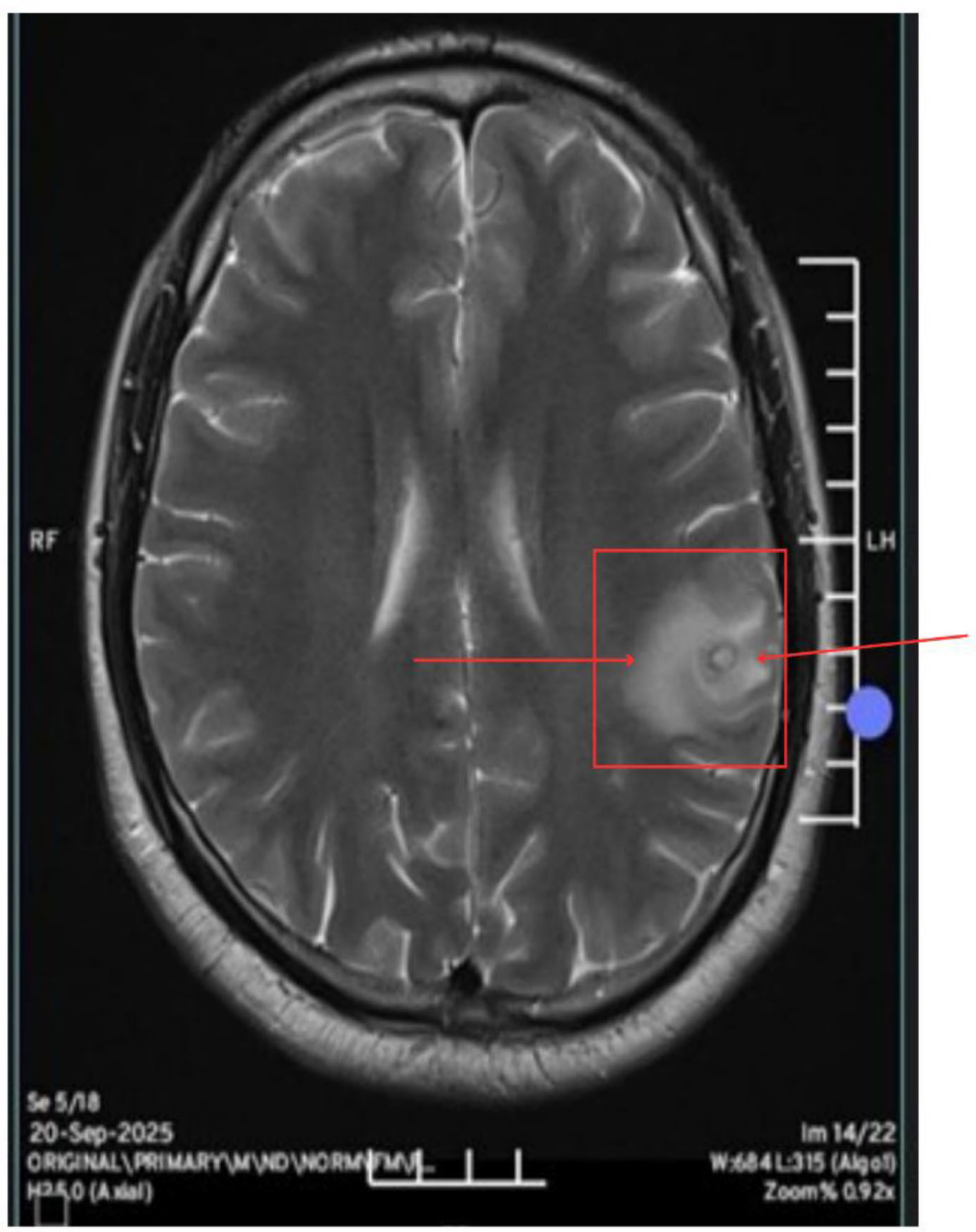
MRI Brain (20 September 2025, performed in India): Axial T2-weighted MRI showing a solitary ring-enhancing lesion with central calcification and mild surrounding edema in the left frontal region, suggestive of neurocysticercosis.
